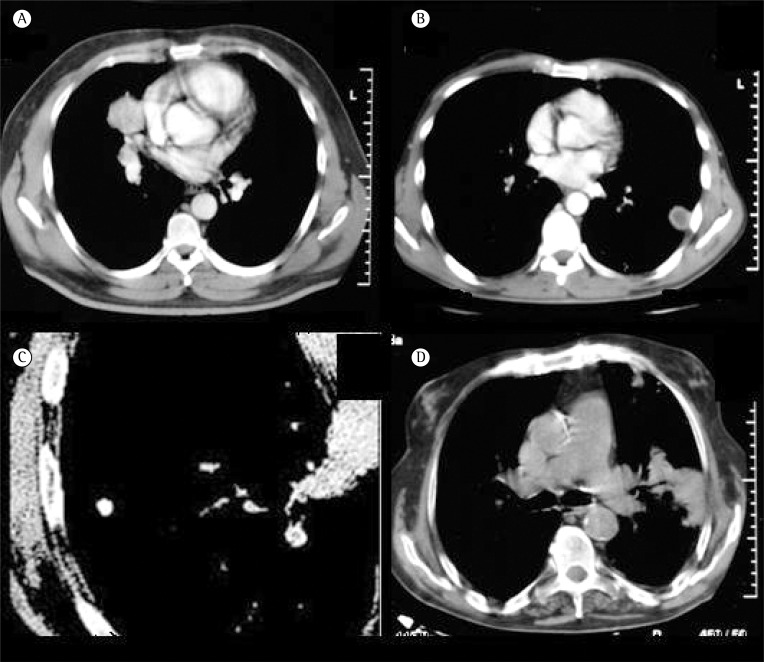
Helical CT scans showing intralesional changes, including homogeneous
